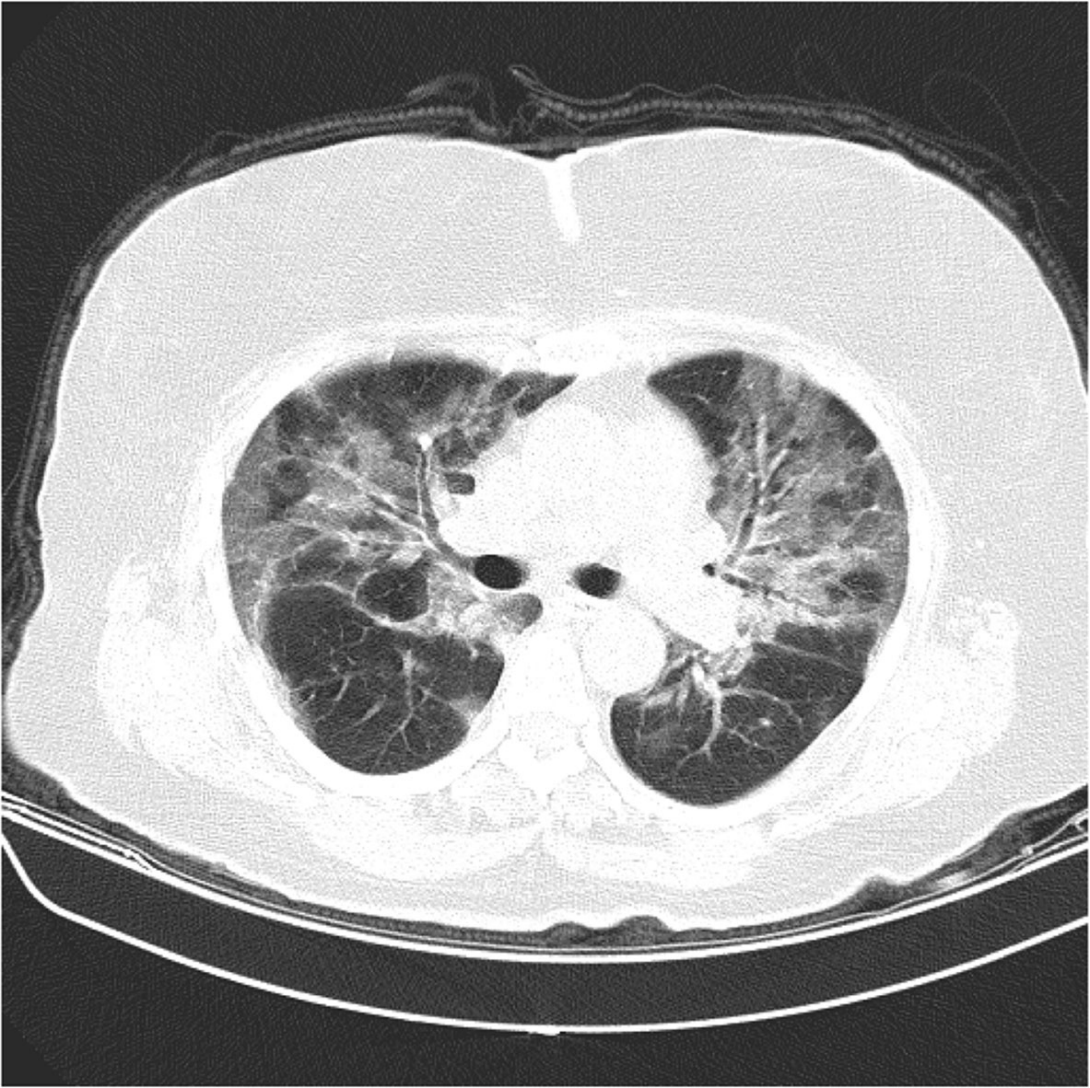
Chest CT scan shows signs of bilateral COVID-19 pneumonia
